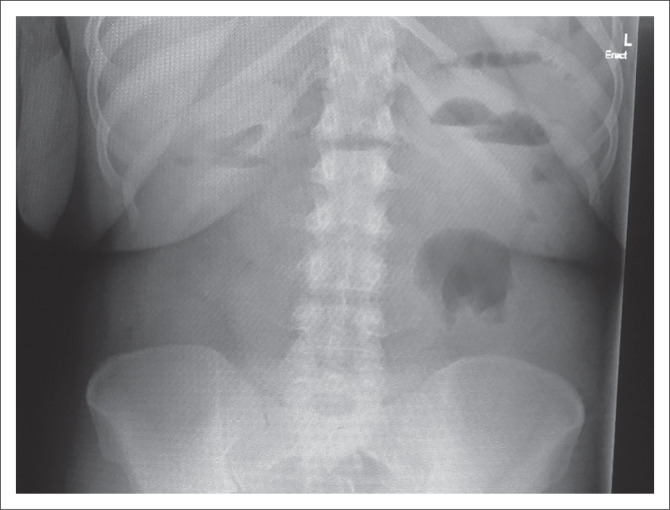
The patient’s erect abdominal radiograph. Multiple air-fluid levels are depicted.
